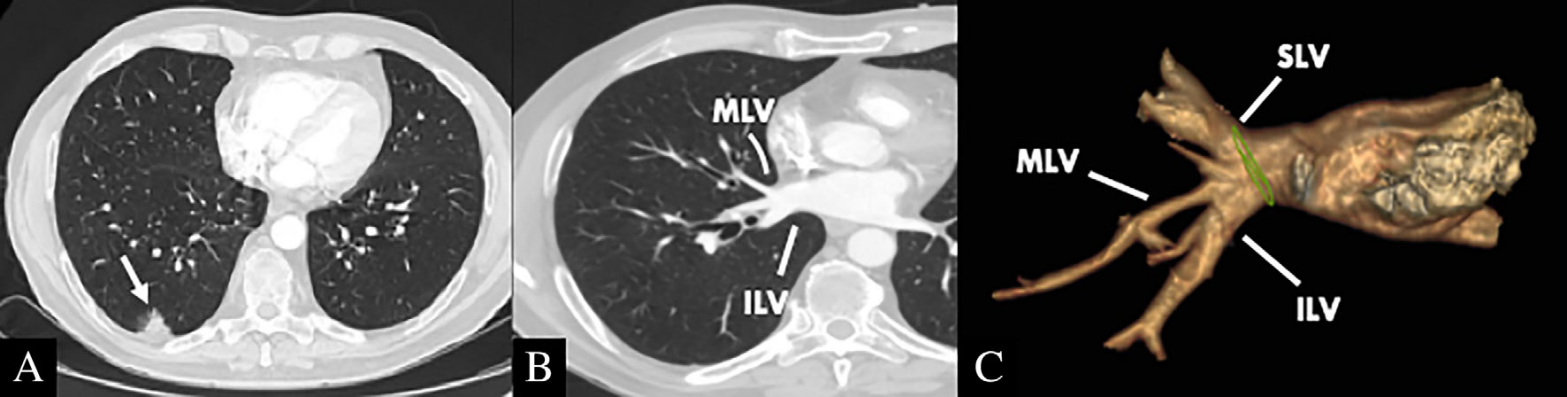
Preoperative enhanced chest CT scans. (A) NSCLC located in the right lower lobe (white arrow). (B) MLV joins the ILV to form the right inferior pulmonary vein. (C) 3D CT reconstruction of right pulmonary veins shows the MLV and ILV forming a single venous confluence. 3D, three‐dimensional; CT, computed tomography; ILV, inferior lobar vein; MLV, middle lobe vein; NSCLC, non‐small cell lung cancer; SLV, superior lobar vein.
